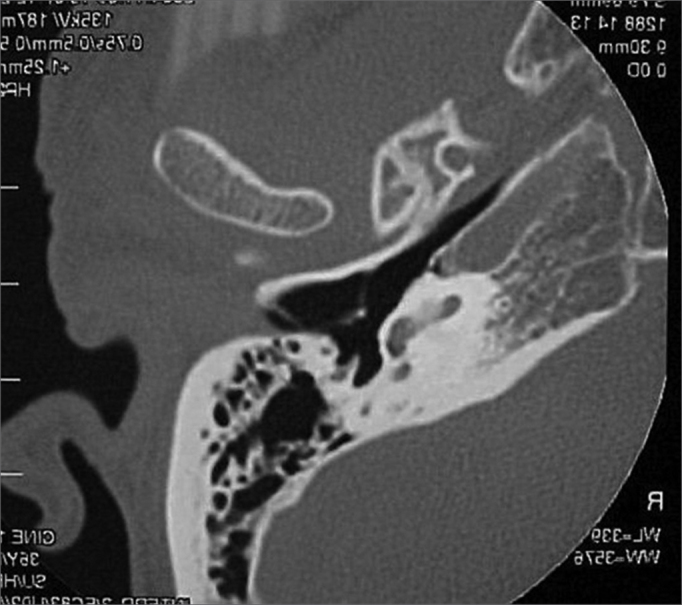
View of the left tube, with the CT scan for comparison.
